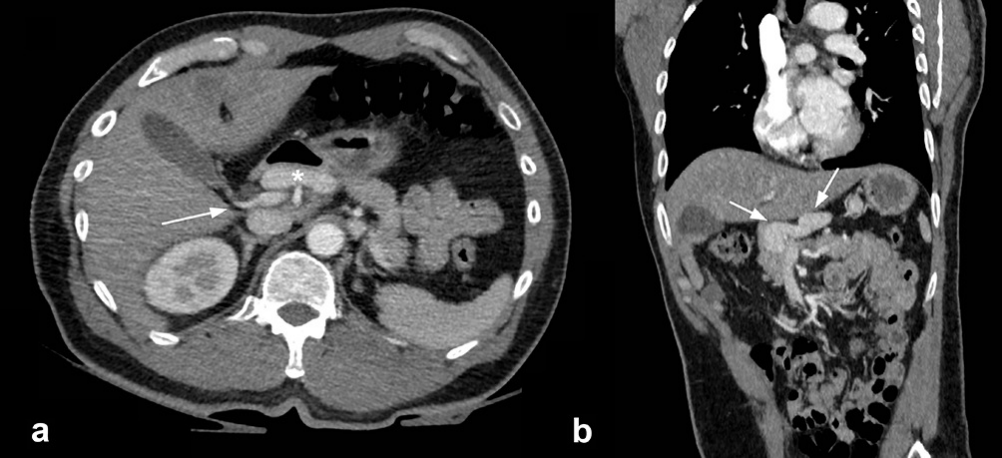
(a) Axial CT image of the upper abdomen (1 mm maximum intensity projection) showing a distended left gastric vein acting as a shunt (asterisk) anterior to the portal vein (arrow), which is seen anterior to the normal size inferior vena cava. (b) Coronal reconstructed CT image (1 mm, maximum intensity projection) showing the shunt joining at the confluence of the superior mesenteric and splenic veins (side-to-side anastomosis) within the upper abdomen, surrounding the head of the pancreas (Supplementary Video 2a,b).
